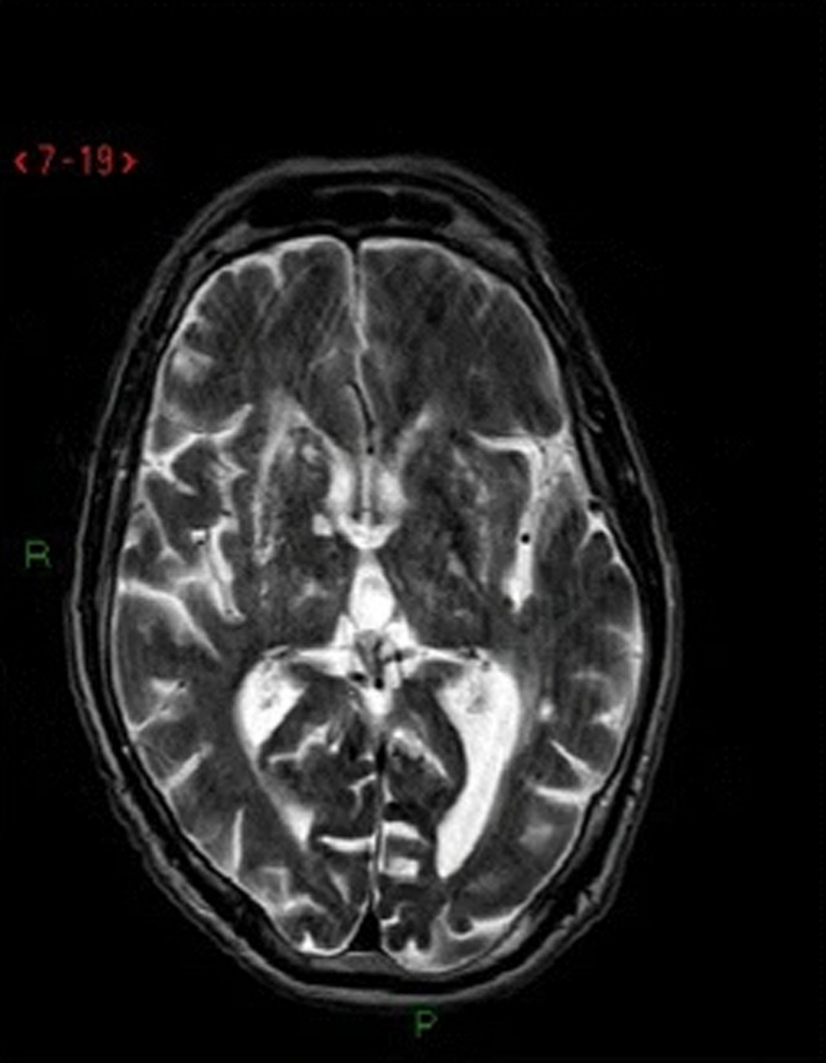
Example of “état crible” on MRI imaging. Reprinted with permission from Pati et al [^23^] Copyright 2018, Springer.
